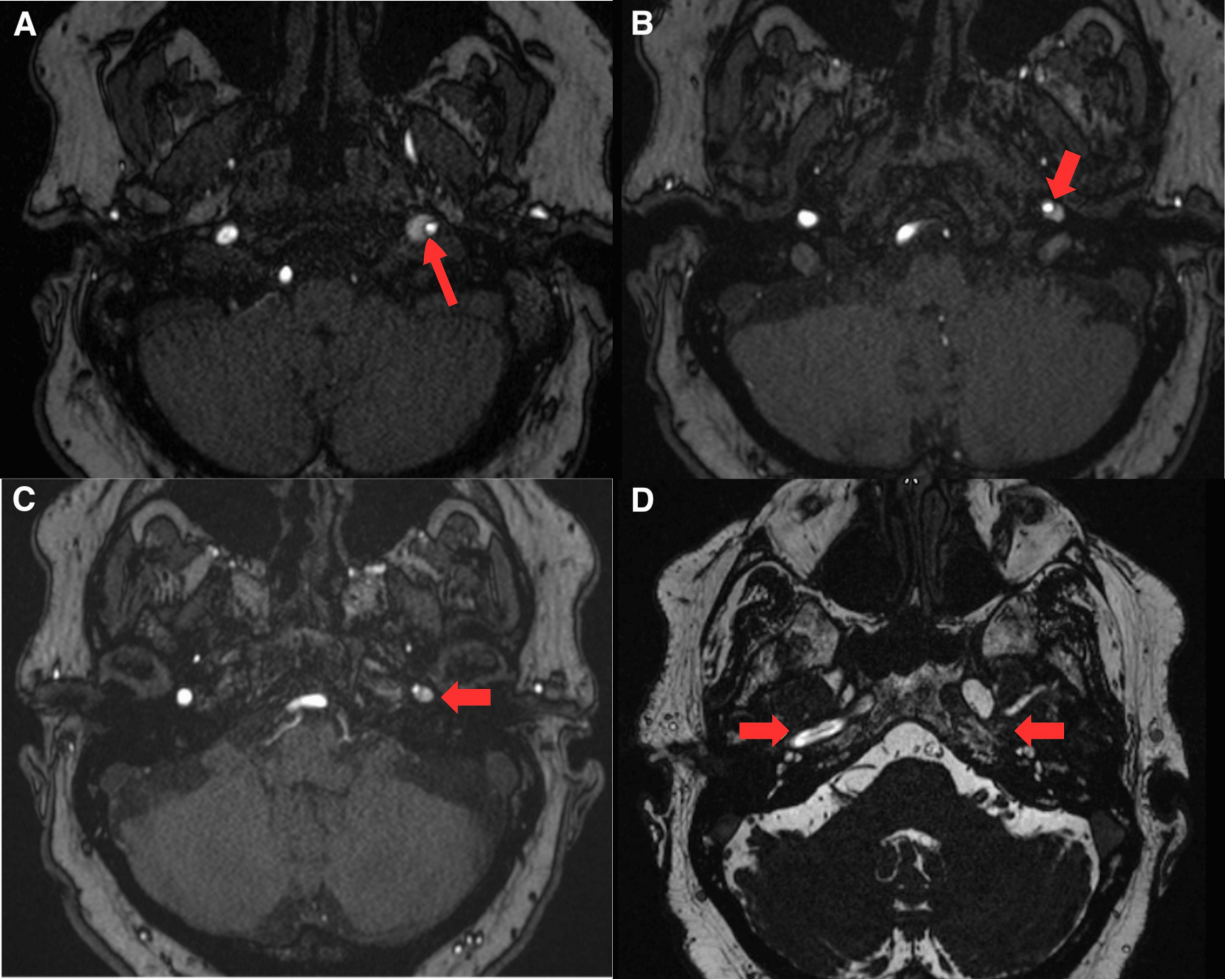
Time-of-flight (TOF) MRI angiography showing dissection of the left ICA in multiple cross-sections from the neck to the skull base (A-C) Demonstrates left internal carotid artery (ICA) in multiple cross-sections at the neck level, and (D) shows the skull base level with a patent right ICA and a stenosed left ICA secondary to dissection.
